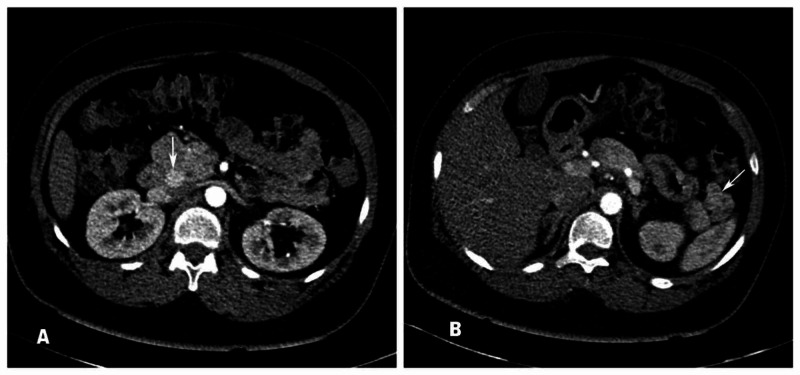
CT images showing pancreatic NET Axial pancreatic phase images of multiphase contrast-enhanced CT images show hyper-enhancing nodule in the pancreatic head (A) and another heterogeneous exophytic lesion with eccentric nodular hyperenhancement in the pancreatic tail region (B) s/o pancreatic neuroendocrine tumor (NET).
